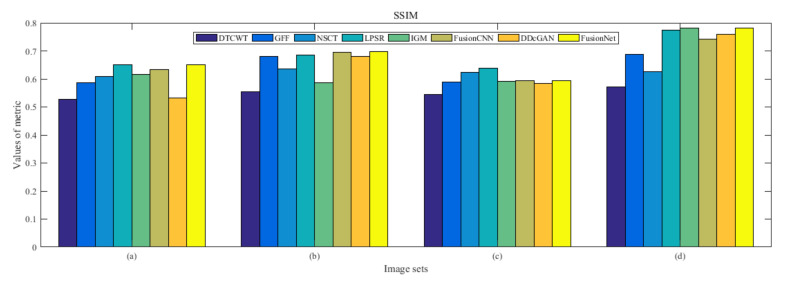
Values of structural similarity (SSIM) in the fused images (MRI-FDG): (a) is composed of eight images in Figure 25, (b) is composed of eight images in Figure 26, (c) is composed of eight images in Figure 27, (d) is composed of eight images in Figure 28.
